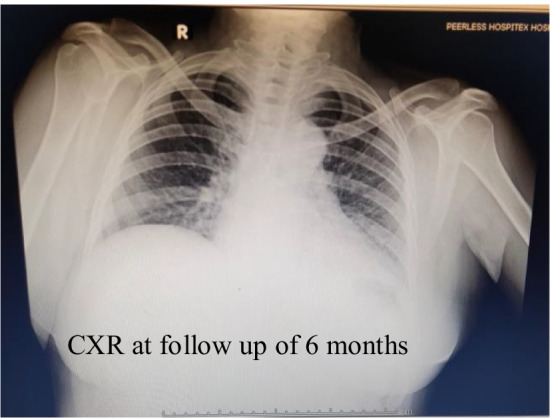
Her chest X-ray (CXR) at a follow-up period of 6 months
